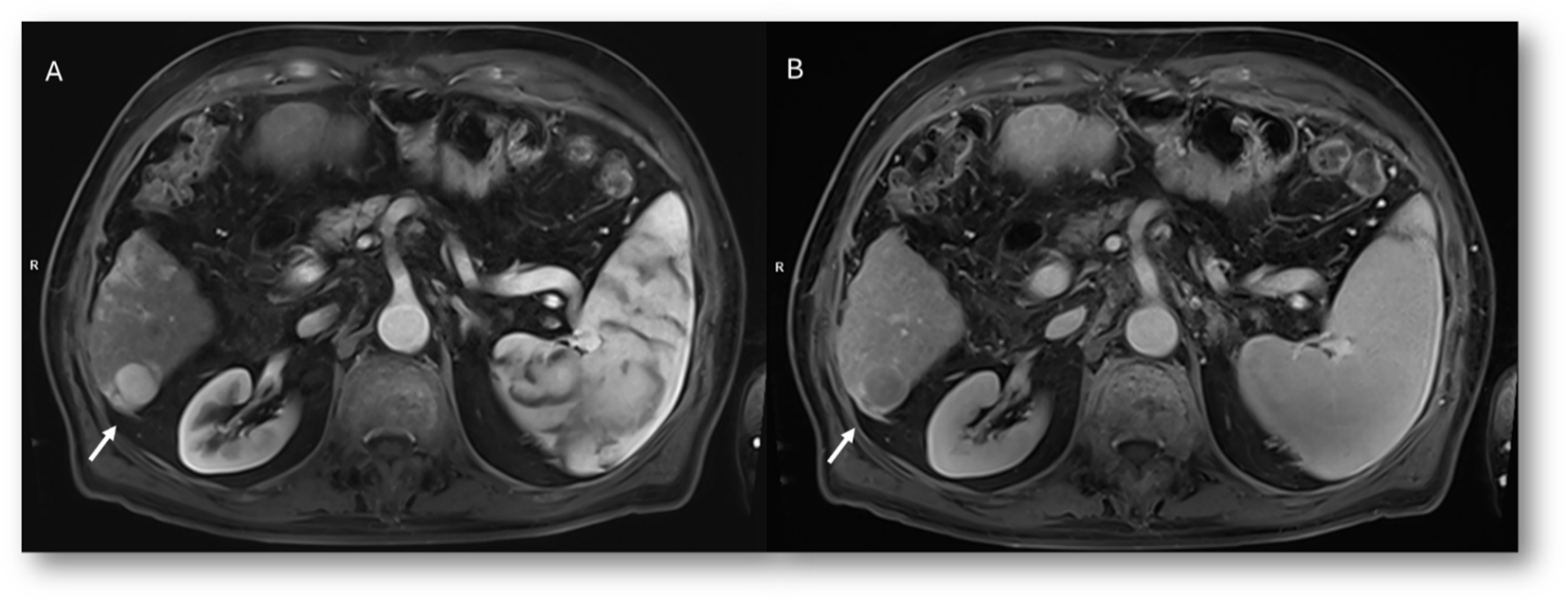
MRI assessment of HCC (arrows). Contrast evaluation with non-specific contrast agent. In (A) and (B) (T1-W FS sequences, axial plane), the lesion shows APHE during arterial phase (A) and washout in portal phase (B), with capsule appearance. These are typical major features according to LI-RADS v2018.
